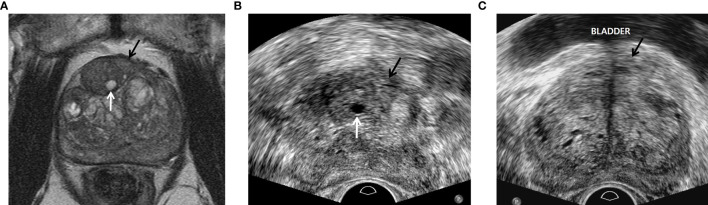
A 77-year-old man with PSA of 9.57 ng/ml. (A) T2-weighted axial magnetic resonance image showing a PI-RADS 4 transition mass (black arrow) in the left base. The tumor is contacting the anterior capsule. The white arrow indicates a small cyst in the neighboring hyperplastic nodule. (B) Transverse TRUS image scanned by radiologist A showing a slightly hyperechoic mass (black arrow) in the left mid-gland. The tumor was targeted with four cores of which three were GS 6 and one was negative. Sextant systematic biopsy was also performed, but all of the cores were negative. The white arrow indicates a small cyst in the neighboring hyperplastic nodule, which is the same imaging feature seen on T2-weighted MR image. This TRUS sequence is fundamental imaging with low dynamic range, resulting in good contrast between the PI-RADS 4 lesion and normal tissue. (C) Transverse TRUS image, which was scanned by radiologist B two months before beginning our study period, shows a slightly hypoechoic mass (black arrow) in the left base contacting the bladder base. The tumor was targeted with three cores of which all were negative. Systematic biopsy was also performed with 10 cores of which all were negative. Radiologist B did not detect the neighboring hyperplastic nodule with a small cyst that was seen on the T2-weighted MR image. This TRUS sequence is harmonic imaging with high dynamic range, subsequently leading to poor tissue contrast.
